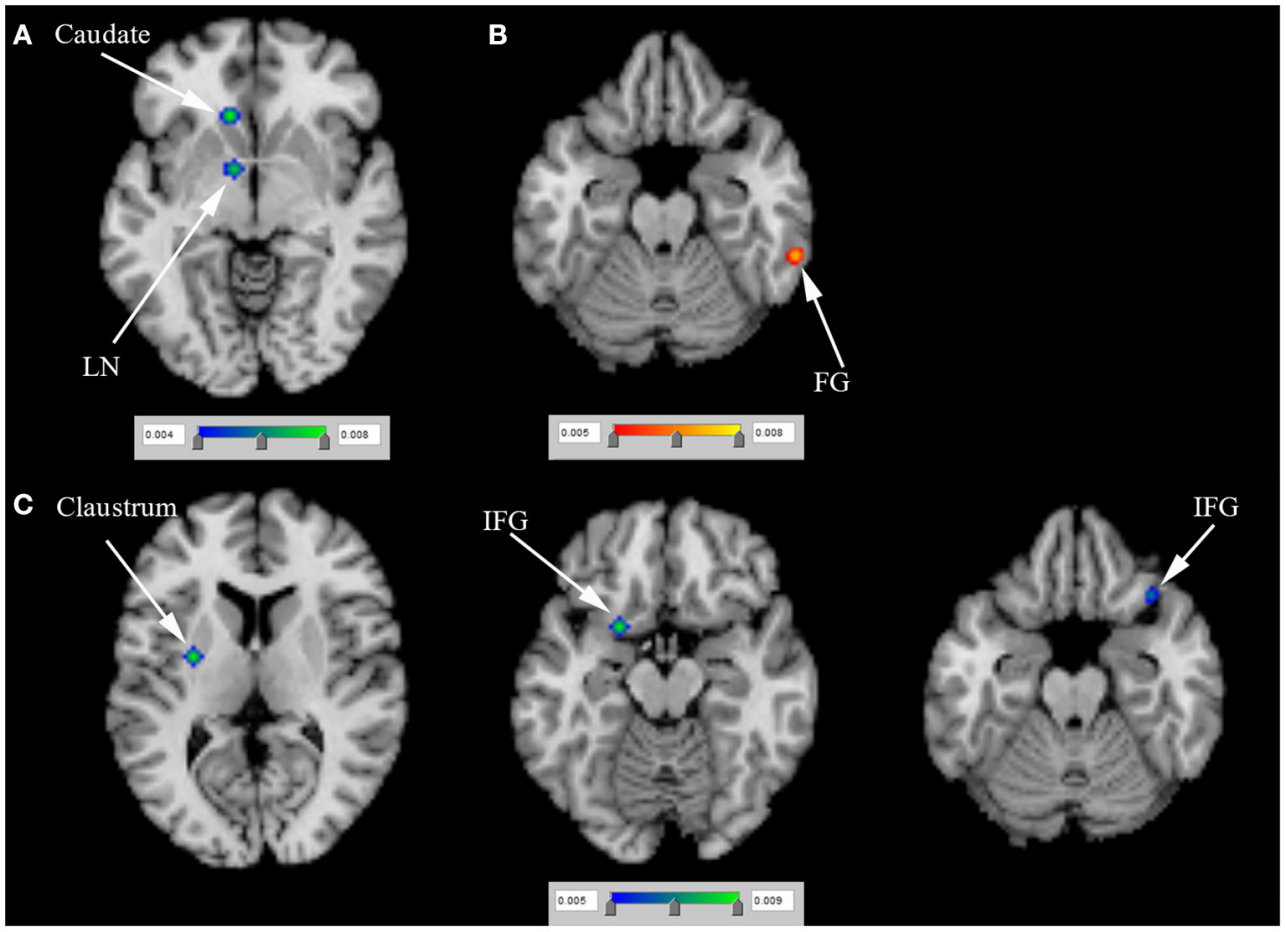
(A) Reduced rCBF (in blue), (B) increased rCBF (in red) using PET and (C) reduced rCBF (in blue) using SPECT in PD-ICD patients, compared to PD-no ICD. FG, fusiform gyrus; ICD, impulse control disorder; IFG, inferior frontal gyrus; LN, lentiform nucleus; PD, Parkinson's disease; PET, positron emission tomography; rCBF, regional cerebral blood flow; SPECT, single photon emission computed tomography.
